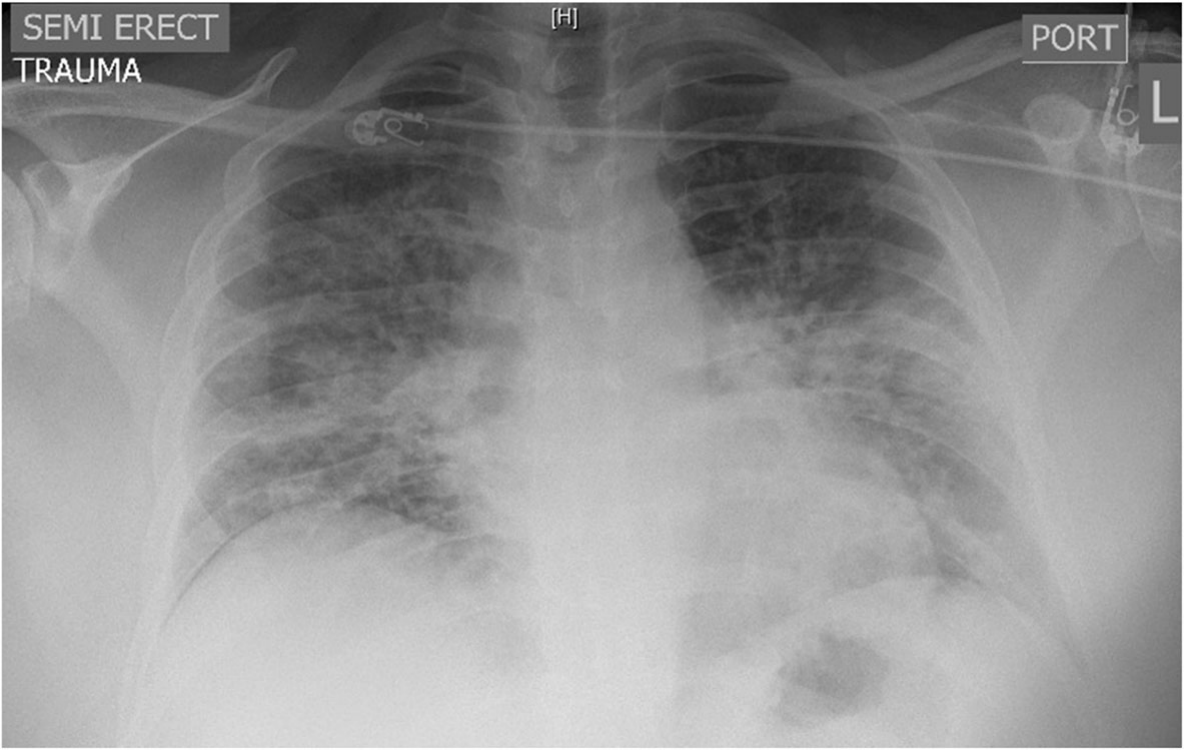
Chest XRay of a patient with EVALI Showing bilateral patchy opacities
